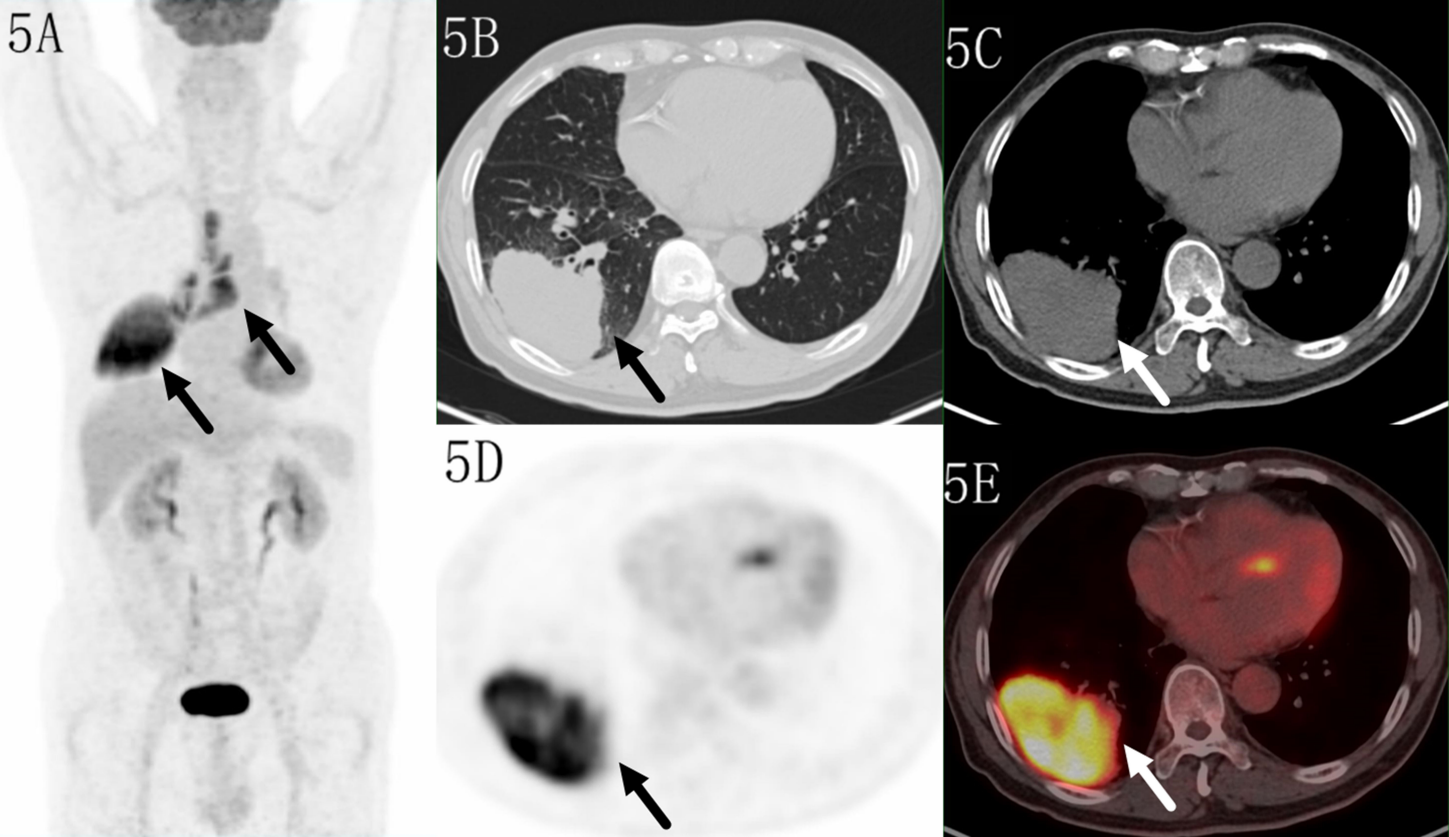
Images of a 70-year-old male patient with adenocarcinoma. PET MIP (A) showed a mass in the right lower lobe and multiple lymph nodes in the right hilar and mediastinum. The lesion located in subpleural site with a SUVmax of 14.4 on transaxialCT (B, C), PET (D) and fused (E) images. Higher uptake was seen in the distal region.
